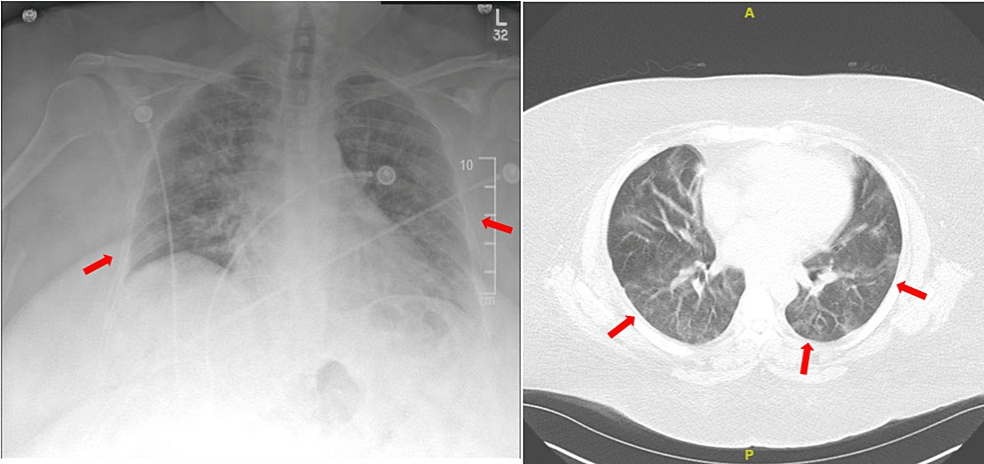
Radiographic imaging including a simple chest x-ray and CT scan showing extensive ground-glass opacities
